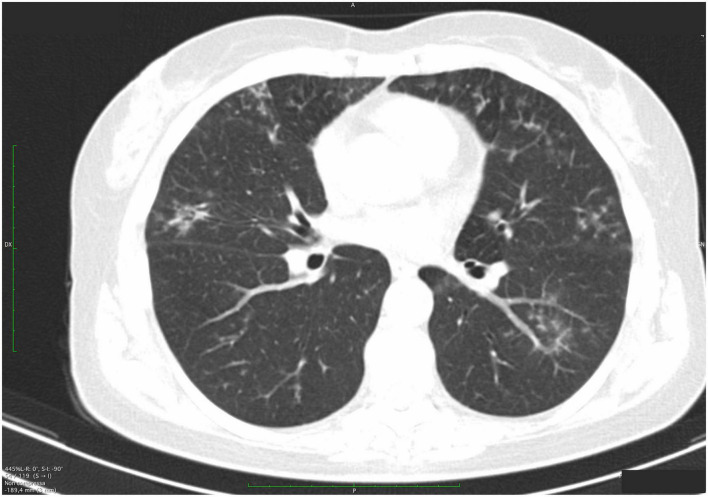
High-resolution computed tomography (HRCT) axial scans of a patient with primary Sjögren’s syndrome (pSS). The patient was a 54-year-old woman with a mild non-productive cough. Multiple semisolid and ill-defined margins centrilobular small nodules are observed, predominantly along the subpleural region, with subpleural interlobular septal thickening. In the right upper lobe, bronchiectasis and early cysts with adjacent peribronchiolar ground-glass thickening are found).
